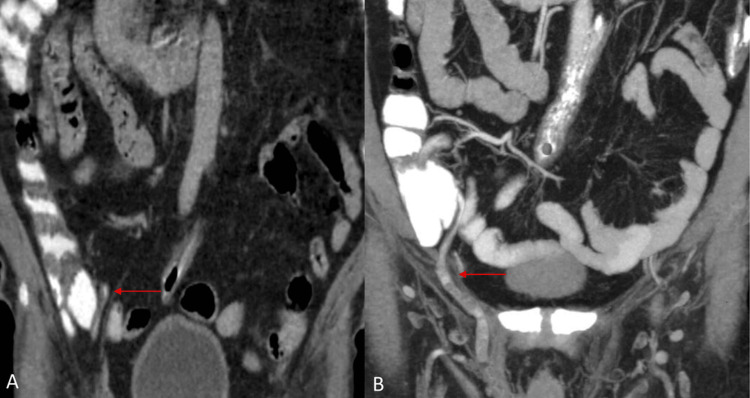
Reformatted coronal CT image showing the appendix arising from the cecum (A, red arrow) entering via the right inguinal hernia (B, red arrow) CT: computed tomography
